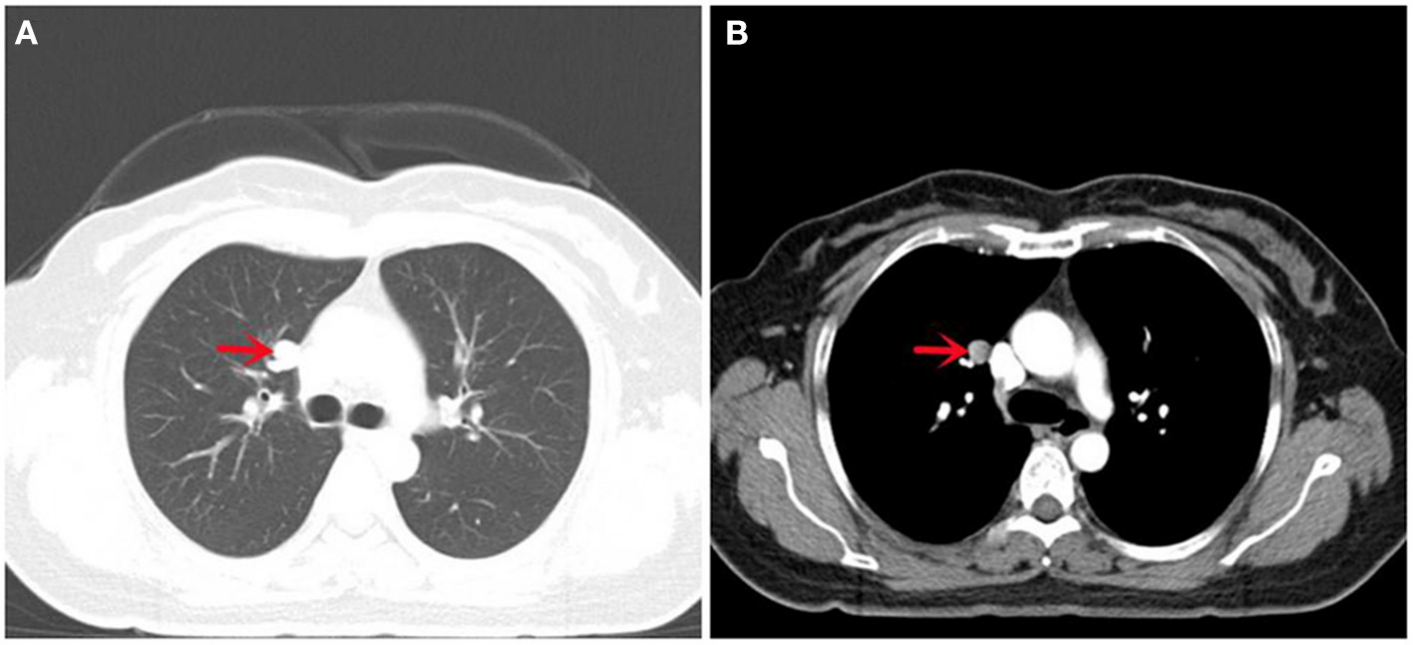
CT images of this case: (A) Lung CT showed a round high-density shadow in the upper lobe of the right lung, with clear boundary and smooth edge, without lobulation and burr; (B) Enhanced CT of the lung showed significant continuous enhancement.
